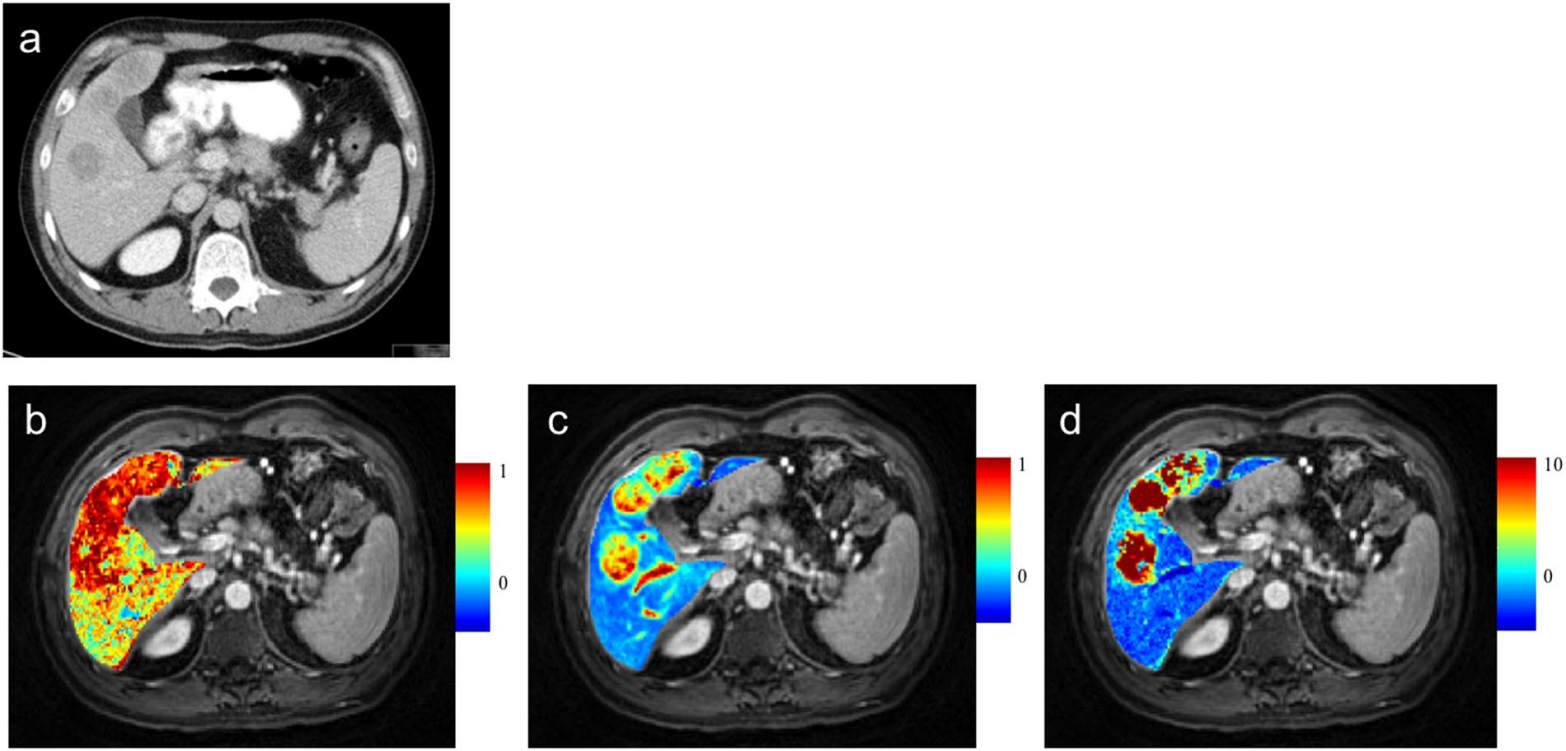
Liver perfusion maps for a patient with metastatic carcinoma lung. Axial CT image shows three hypodense lesions (a). Corresponding liver perfusion maps depict arterial fraction (b), distribution volume (c) and mean transit time (d).
